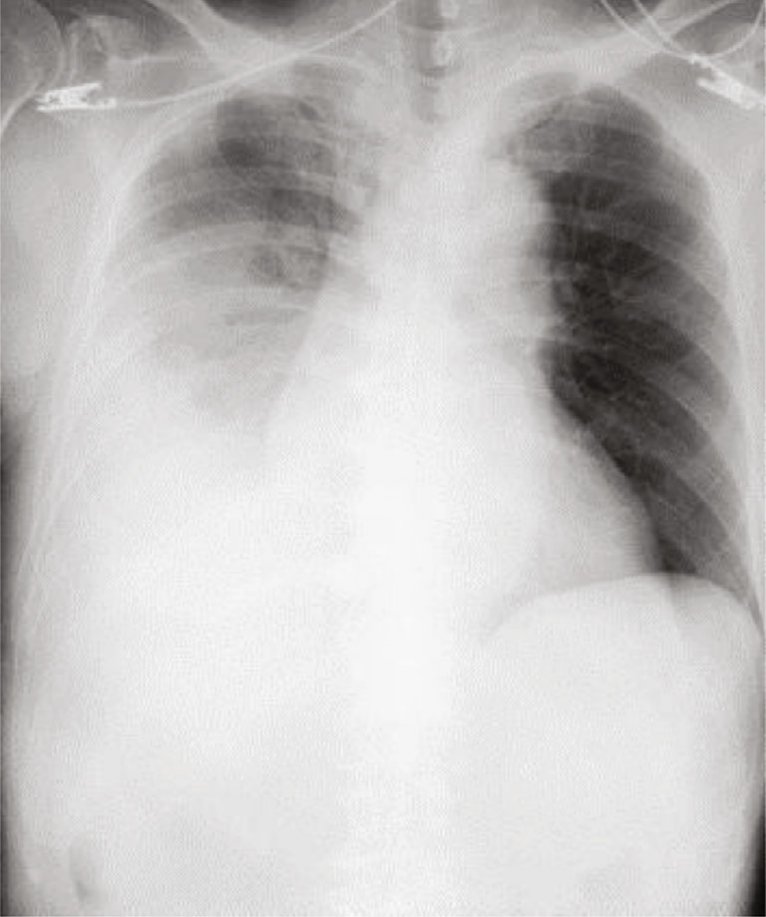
Chest X-ray findings on arrival. Chest X-ray showed reduced permeability of the right lung field.
